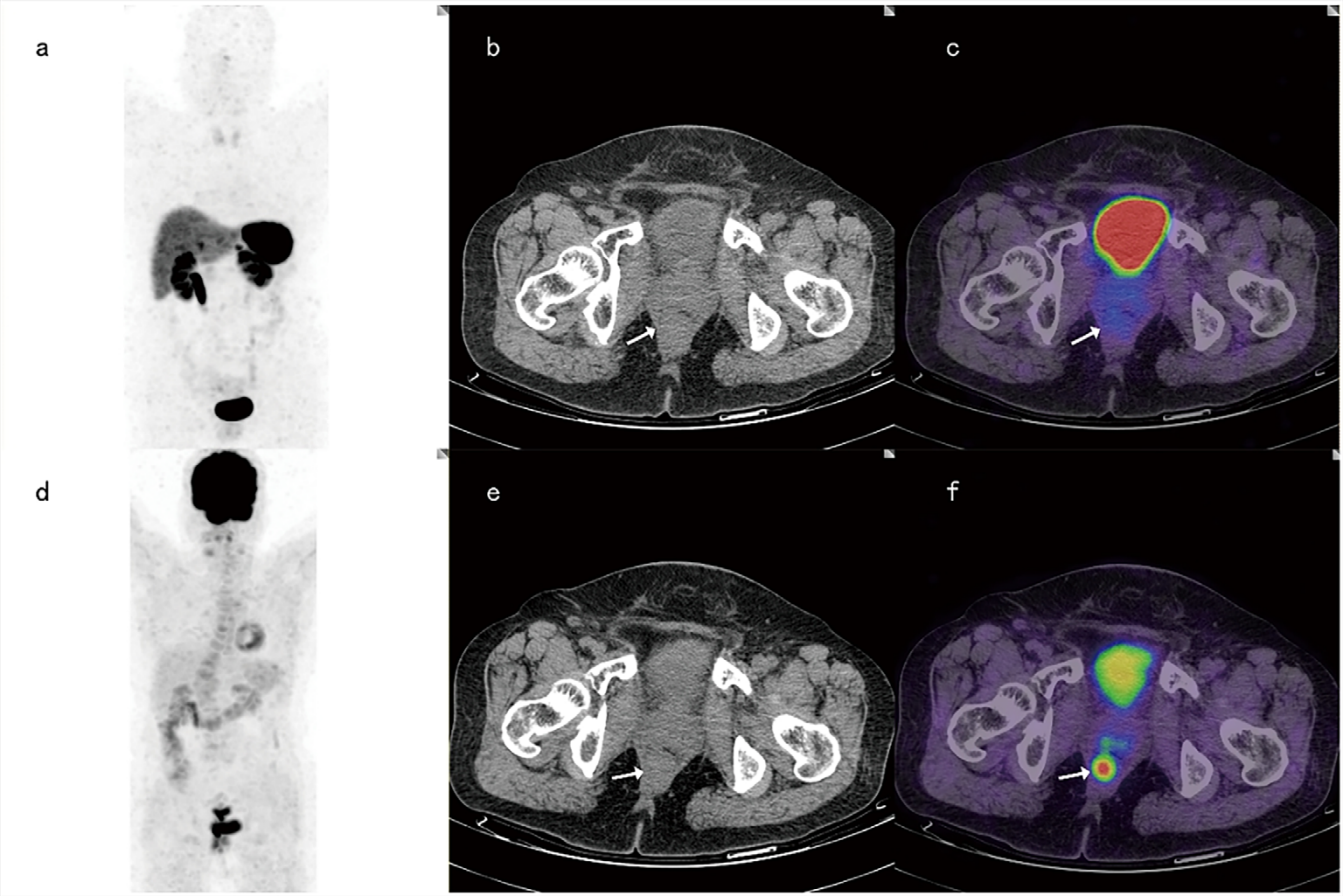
A 65-year-old female patient with rectal neuroendocrine carcinoma (Ki-67 was 90%). 68Ga-DOTANOC PET-CT images and corresponding MIP images (A–C) showed no focal uptake of 68Ga-DOTANOC, while 18F-FDG PET-CT imaging (D–F) showed focal uptake of 18F-FDG.
